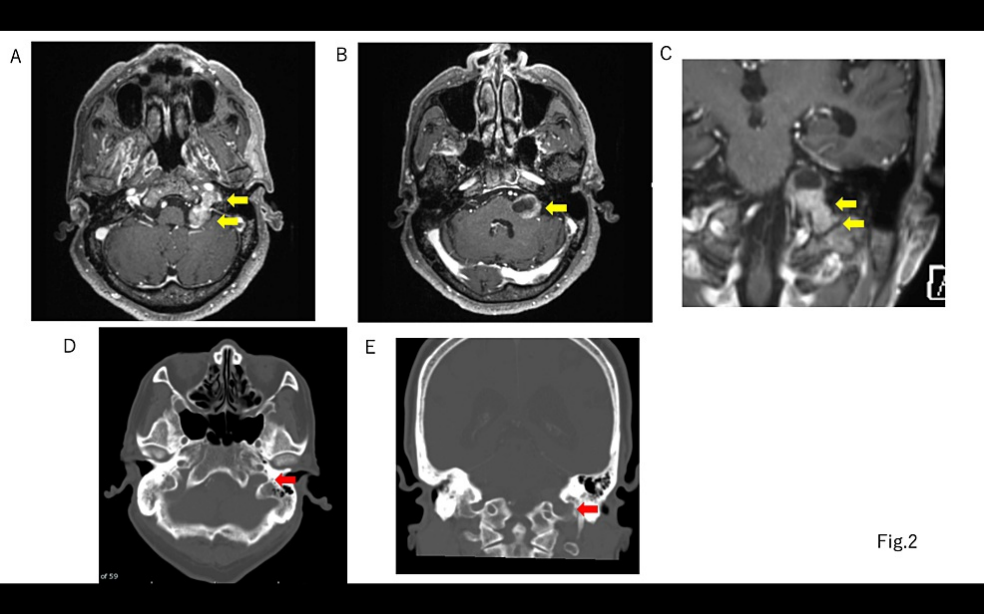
Preoperative gadolinium (Gd) MRI and CT bone images A, B: the tumor was an intracranial and extracranial dumbbell type with an inner cyst. C: Gd MRI coronal image. D: axial plane CT bone image. E: coronal plane CT bone image. Yellow block arrow: jugular foramen tumor. Red block arrow: enlarged jugular foramen CT: computed tomography; MRI: magnetic resonance imaging
